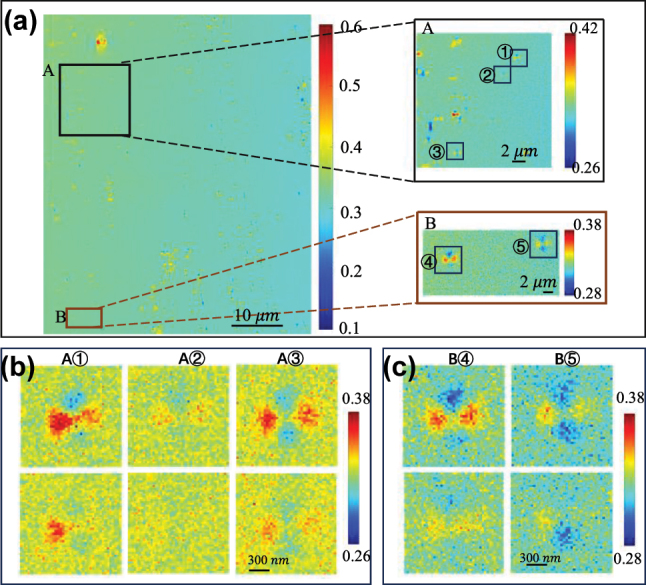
PIMI images (sin*δ*) for the experiment. (a) PIMI images (sin*δ*) obtained by using a 100× objective lens under bright field illumination under the influence of ultrasound in the horizontal direction. The right-hand sides display the magnified outcomes of the left square regions in order. (b) and (c) PIMI images (sin*δ*) for five individual GN in (a) respectively, from top to bottom: under the influence of ultrasound (case E.h.w), and the second row is without ultrasound (case E.h.n).
